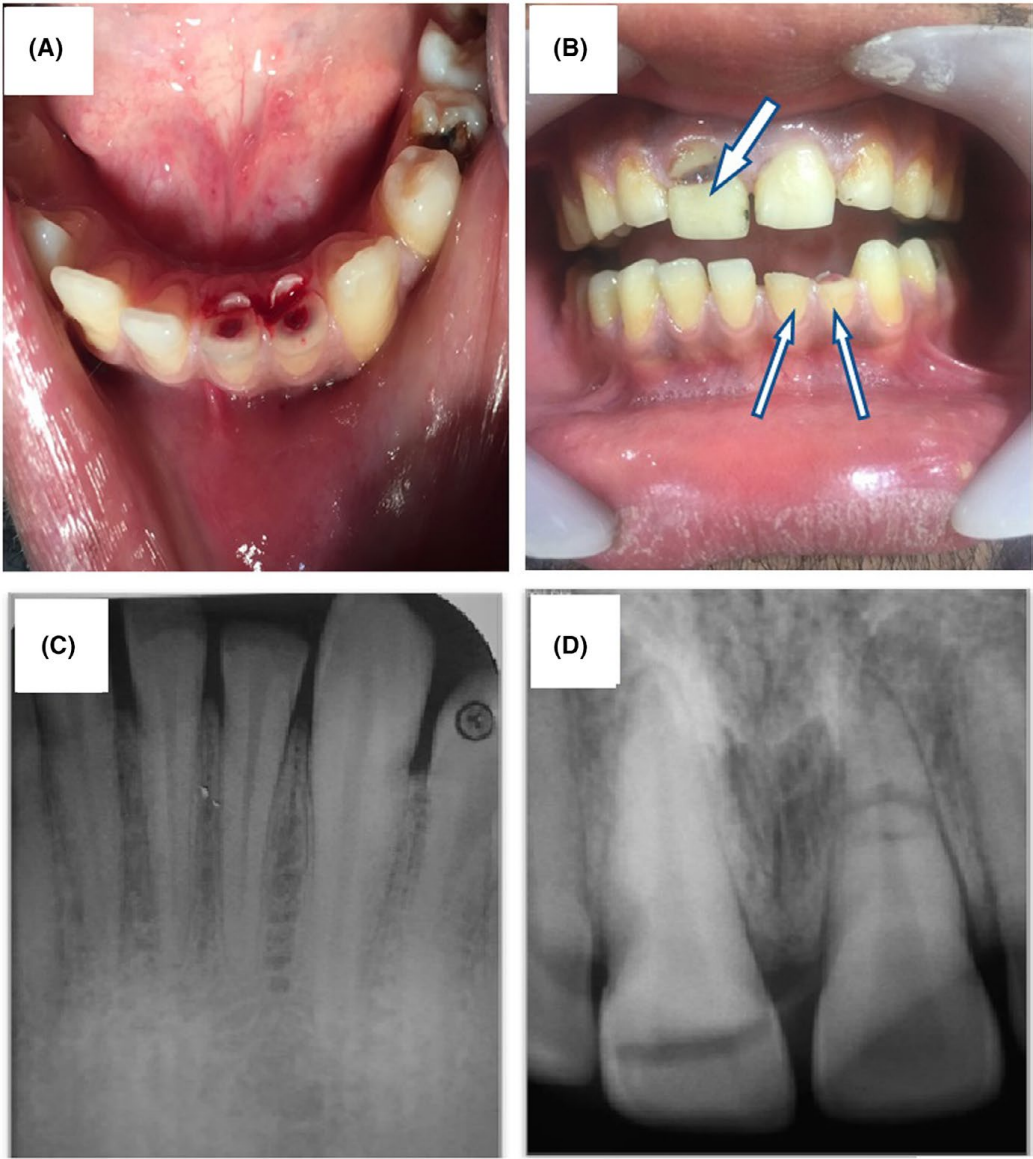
Pre‐operative intra oral pictures (A, B) and periapical radiographs (C, D). The arrows show traumatized teeth
